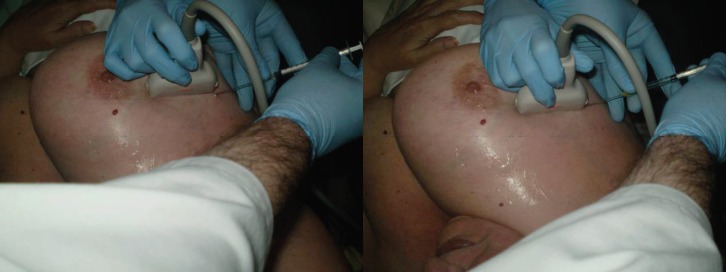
SNOLL technique, ultrasonographic guidance.
